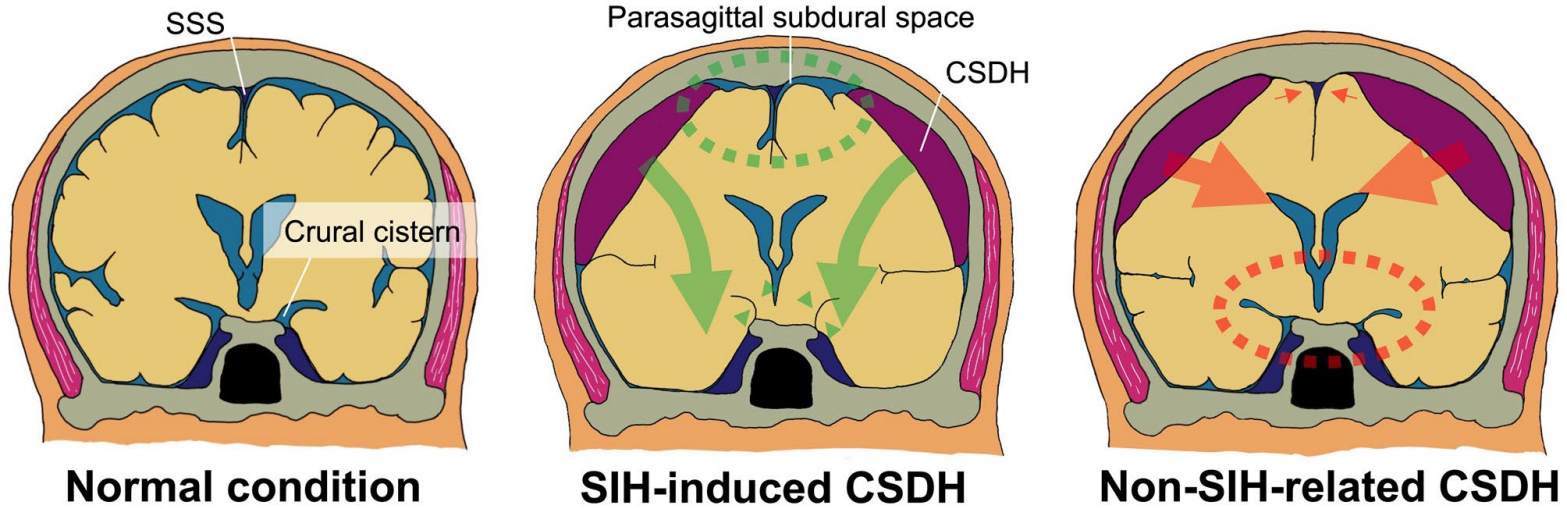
Possible CT-features of SIH-induced and non-SIH-related bilateral CSDH. (Left) Normal condition. PSS (beside SSS) and crural cistern are patent. (Middle) Since bilateral CSDH occurs due to CSF leakage at skull-base or spinal level (green thick-arrows) in SIH-induced case, crural cistern is obliterated (green arrowheads), and PSS is patent (green dotted area). (Right) On the contrary, in non-SIH-related bilateral CSDH, crural cistern is patent (orange dotted area), and PSS is obliterated (orange small-arrows) as hematoma compresses the supratentorial components in midline (orange thick-arrows). SIH: spontaneous intracranial hypotension syndrome, CSDH: chronic subdural hematoma, SSS: superior sagittal sinus
